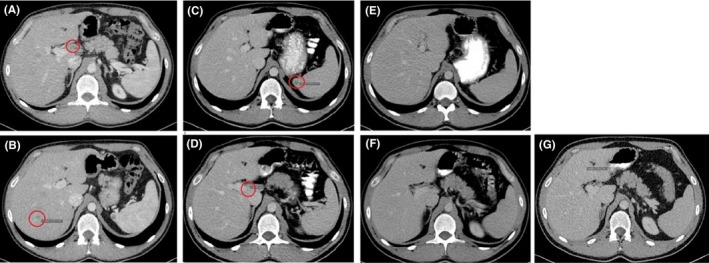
Abdominal contrast‐enhanced CT late phase scans at 6.6 mo show (A) a hypovascular focal lesion in the right lobe of the liver of up to 1 cm in diameter and (B) in the spleen up to 1 cm. (C, D) In the scan at 10.2 mo, the lesion in right liver lobe of the liver had disappeared and the lymph node in the hepatic hilus has been reduced in size. (E, F) At 1.3 y and (G) at 1.5 y, there is no change in size of the lymph nodes and lesions in the liver are not observed
